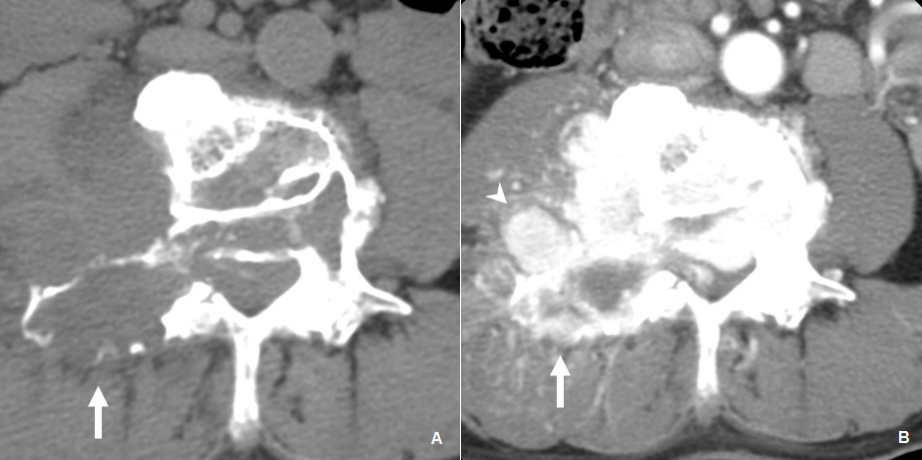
Pre (left) and arterial phase (right) axial CT images showing the recurrent haemangioblastoma with intradural and osseous extension. Arterial contrast enhancement is present in the hypervascular extradural components (arrowhead). There is bony erosion of the right pedicle and transverse process by the hypervascular tumour (solid arrow).
